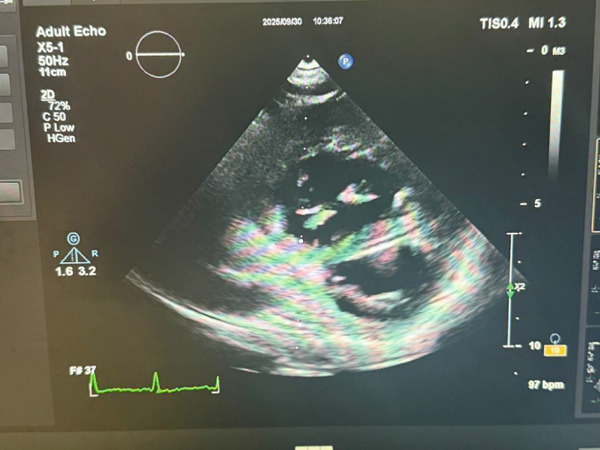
Patient′s transthoracic echocardiography finding.
